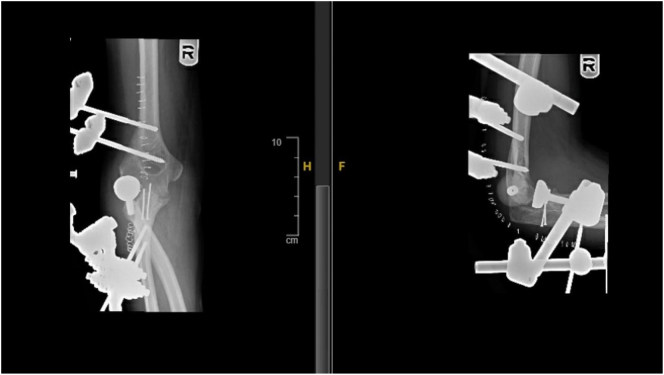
X-ray showing the external fixators and aligning of the radial head prosthesis.
